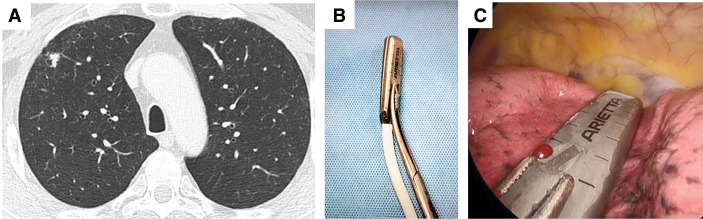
Preoperative and intraoperative findings. (A) Preoperative CT image showing a 1.1-cm nodule in the apical segment (S1) of the right lung, located 0.9 cm away from the pleural surface. (B) The back of the probe was grasped with forceps. (C) Intraoperative ultrasound with a probe placed directly on the lung surface.
